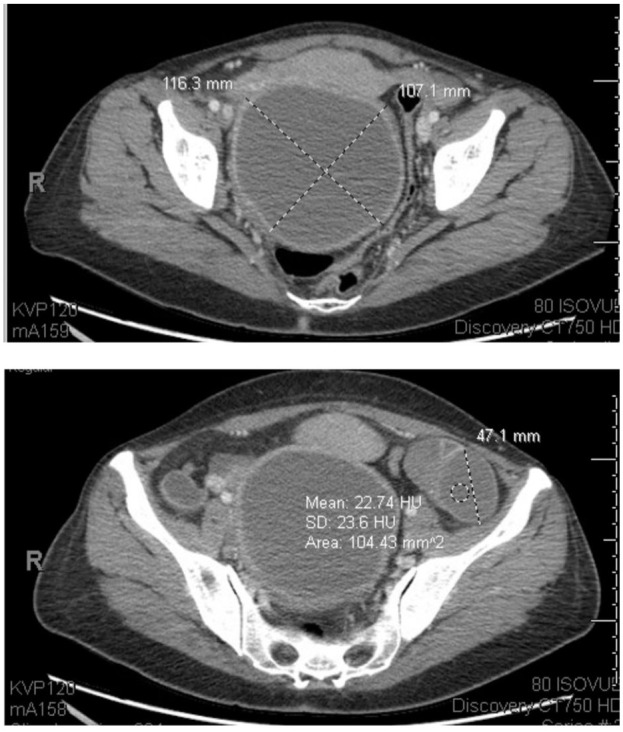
Second ED presentation workup: CT abdomen pelvis showing increased right adnexal cystic lesion with a thick enhancing wall measuring 11.6 cm × 10.7 cm × 10 cm compared to 10 cm × 8.7 cm × 8.6 cm during initial presentation. Multiple left ovarian hypoattenuating lesions, the largest measuring 4.7 cm, new compared to prior exam.
